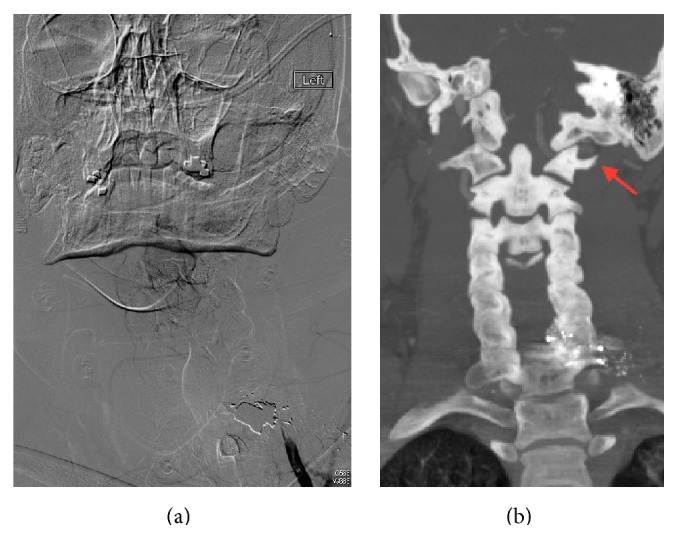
(a) Angiography showing occlusion of the left vertebral artery. (b) CT angiogram showing reconstitution of the left vertebral artery (red arrow).
